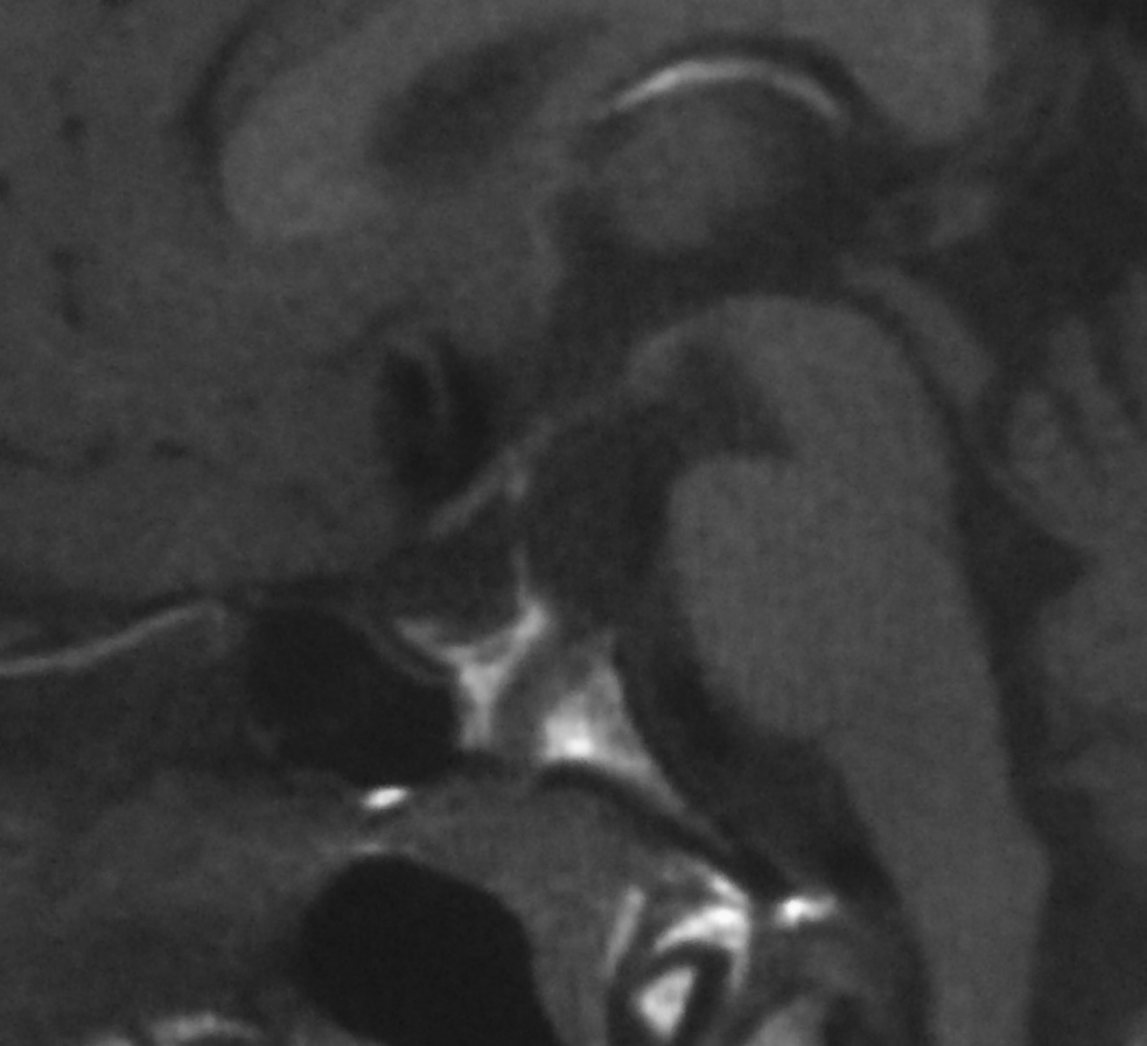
Magnetic resonance imaging (MRI) image from the patient’s mother. Her pituitary MRI showed a thin pituitary stalk and hypoplasia of the adenohypophysis
